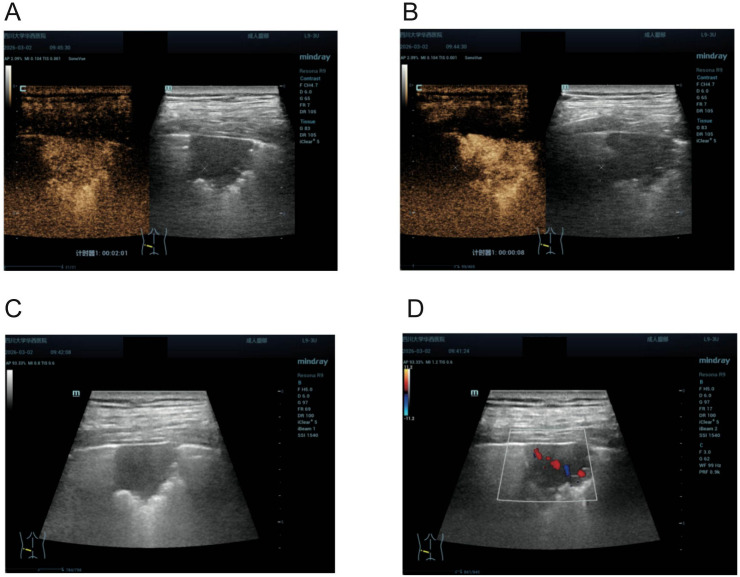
Multimodal ultrasonographic findings of the left lower lobe lesion. (A) CEUS shows relatively homogeneous internal enhancement with slight persistent hyperenhancement in the venous phase, favoring an inflammatory lesion. (B) CEUS shows hyperenhancement in the pulmonary arterial phase. (C) Gray-scale ultrasonography shows a hypoechoic subpleural lesion in the dorsal left lower lobe, measuring approximately 2.4 × 2.2 cm. (D) Color Doppler imaging shows marked intralesional vascularity, with obvious blood-flow signals detected within the lesion.
